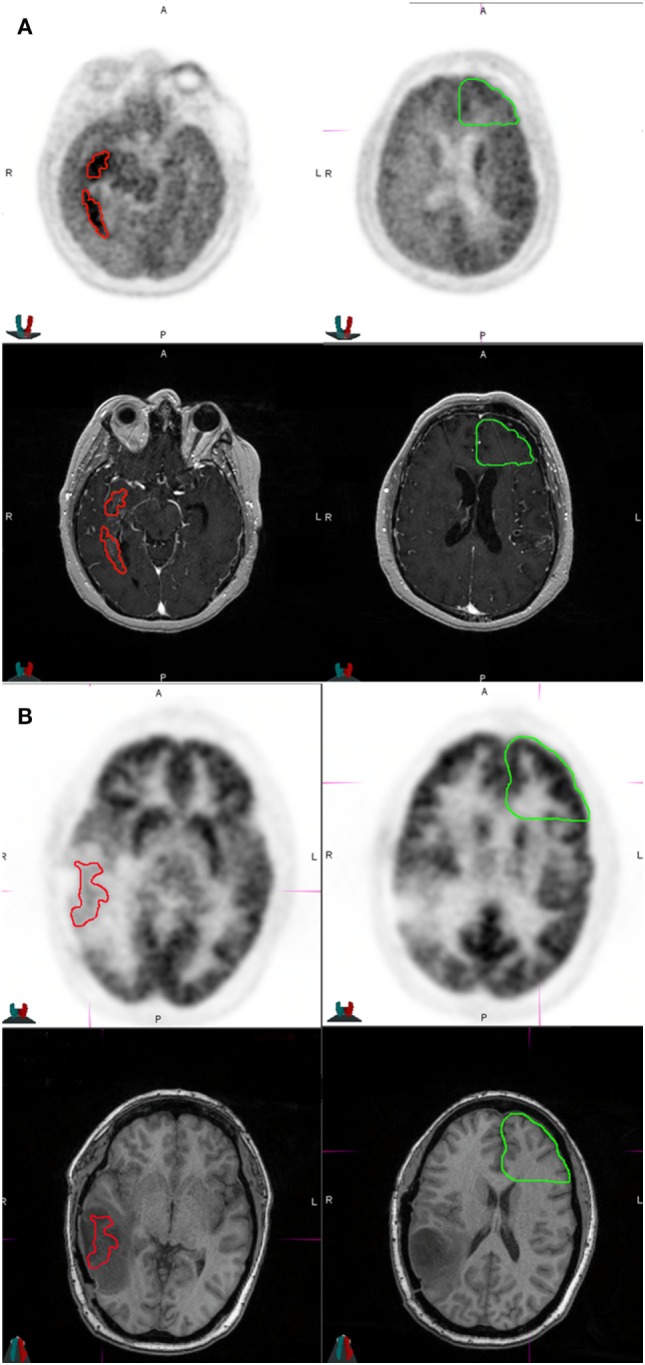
Representative patients in the study who were classified as high risk and low risk at the time of imaging. (A) The patient was a 36-year-old male who had MGMT non-methylated, isocitrate dehydrogenase 1 (IDH1) wild-type multifocial glioblastoma multiforme of the right temporal lobe at diagnosis, and received biopsy, radiation therapy to 63 Gy with concurrent temozolomide, followed by adjuvant temozolomide and bevacizumab therapy for 13 months prior to this MRI and [^18^F]fluorodeoxyglucose (FDG)-positron emission tomography (PET) scan. The tumor had a SUV_max_ of 11.64, a SUV_max_/NB ratio of 2.00, and an apparent diffusion coefficient (ADC) of 1,031 × 10^−6^ mm^2^/s. He was classified as high risk and treated with erlotinib. He developed further progression on imaging after 1 month. This patient died of disease at 2 months after this FDG-PET scan. The top two panels show axial slices of the FDG-PET scan at the level of the tumor (left) and normal brain (right). The bottom two panels show axial slices of the T1 contrast image at the level of the tumor (left) and normal brain (right). Red represents tumor region of interest used for deriving imaging parameters. Green represents contralateral uninvolved gray matter for normalizing SUV_max_. (B) The patient is a 37-year-old female who had MGMT methylated, IDH1-mutated glioblastoma multiforme of the left temporal lobe treated with gross total resection, radiation therapy to 60 Gy with concurrent temozolomide, followed by adjuvant temozolomide with a tumor treating fields device (Novocure Optune) for 5 months prior to this MRI and FDG-PET scan. The imaging showed an SUV max of 8.6, SUV_max_/NB ratio of 0.60, and an ADC of 1,987 × 10^−6^ mm^2^/s. She was classified as low risk and continued treatment with tumor treating fields device. She did not progress after 6 months and was classified as having treatment effect. She is alive and without progression of disease at last follow-up 13 months after this FDG-PET scan. The top two panels show axial slices of the FDG-PET scan at the level of the tumor (left) and normal brain (right). The bottom two panels show axial slices of the fluid attenuation inversion recovery at the level of the tumor (left) and normal brain (right).
